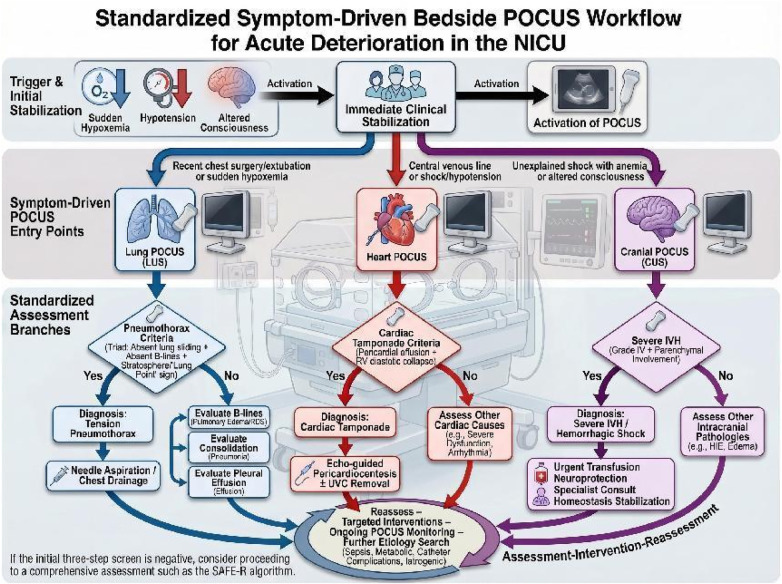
A structured POCUS screening pathway for the crashing neonate in the NICU. The algorithm outlines a systematic, bedside approach involving rapid sequential examination of the lungs, heart, and brain to expedite the diagnosis or exclusion of critical conditions: tension pneumothorax, pericardial tamponade, and severe IVH. LUS, lung ultrasound; CUS, cranial ultrasound.
